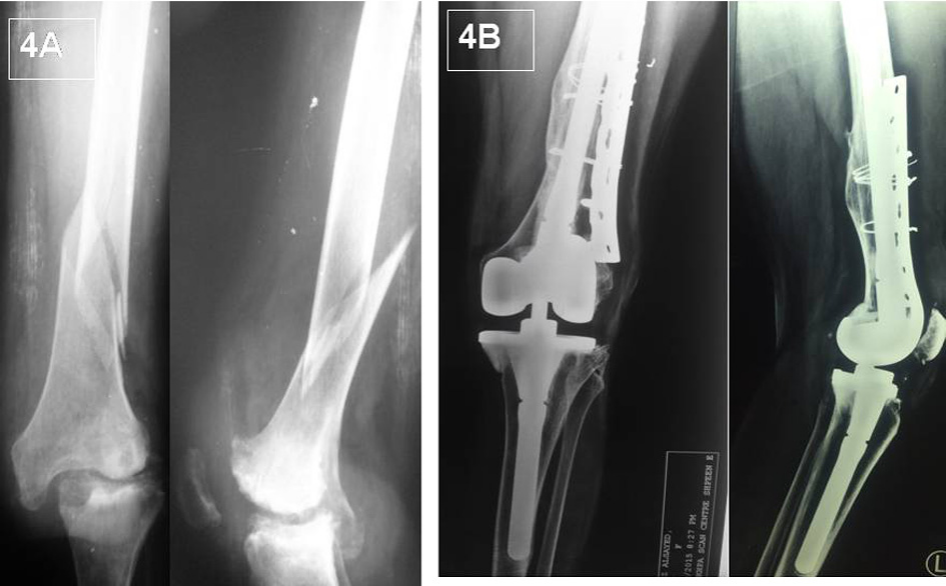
(A) Preoperative X-rays for a 63 rheumatoid lady with advanced knee arthritis and acute distal femoral fracture. (B) The strategy of fix and replace was followed with simultaneous fixation of the fracture and replacement of the knee using an LCCK implant. 7 years follow up with full union of the fracture and stable functioning knee prothesis.
